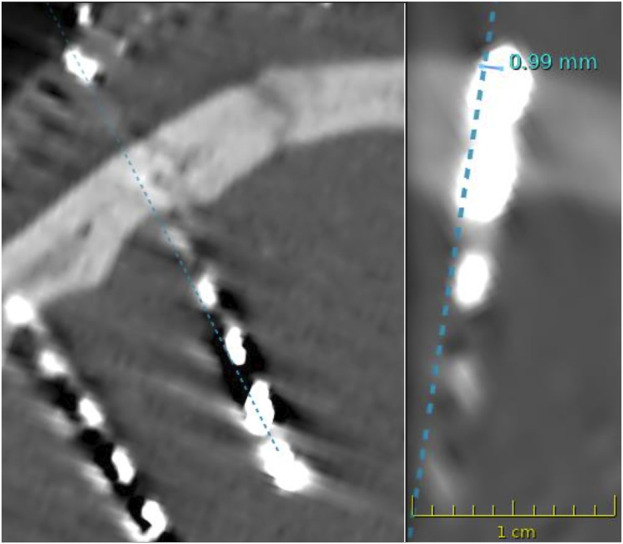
Postoperative computed tomography imaging showing entry point deviations of < 1 mm.
